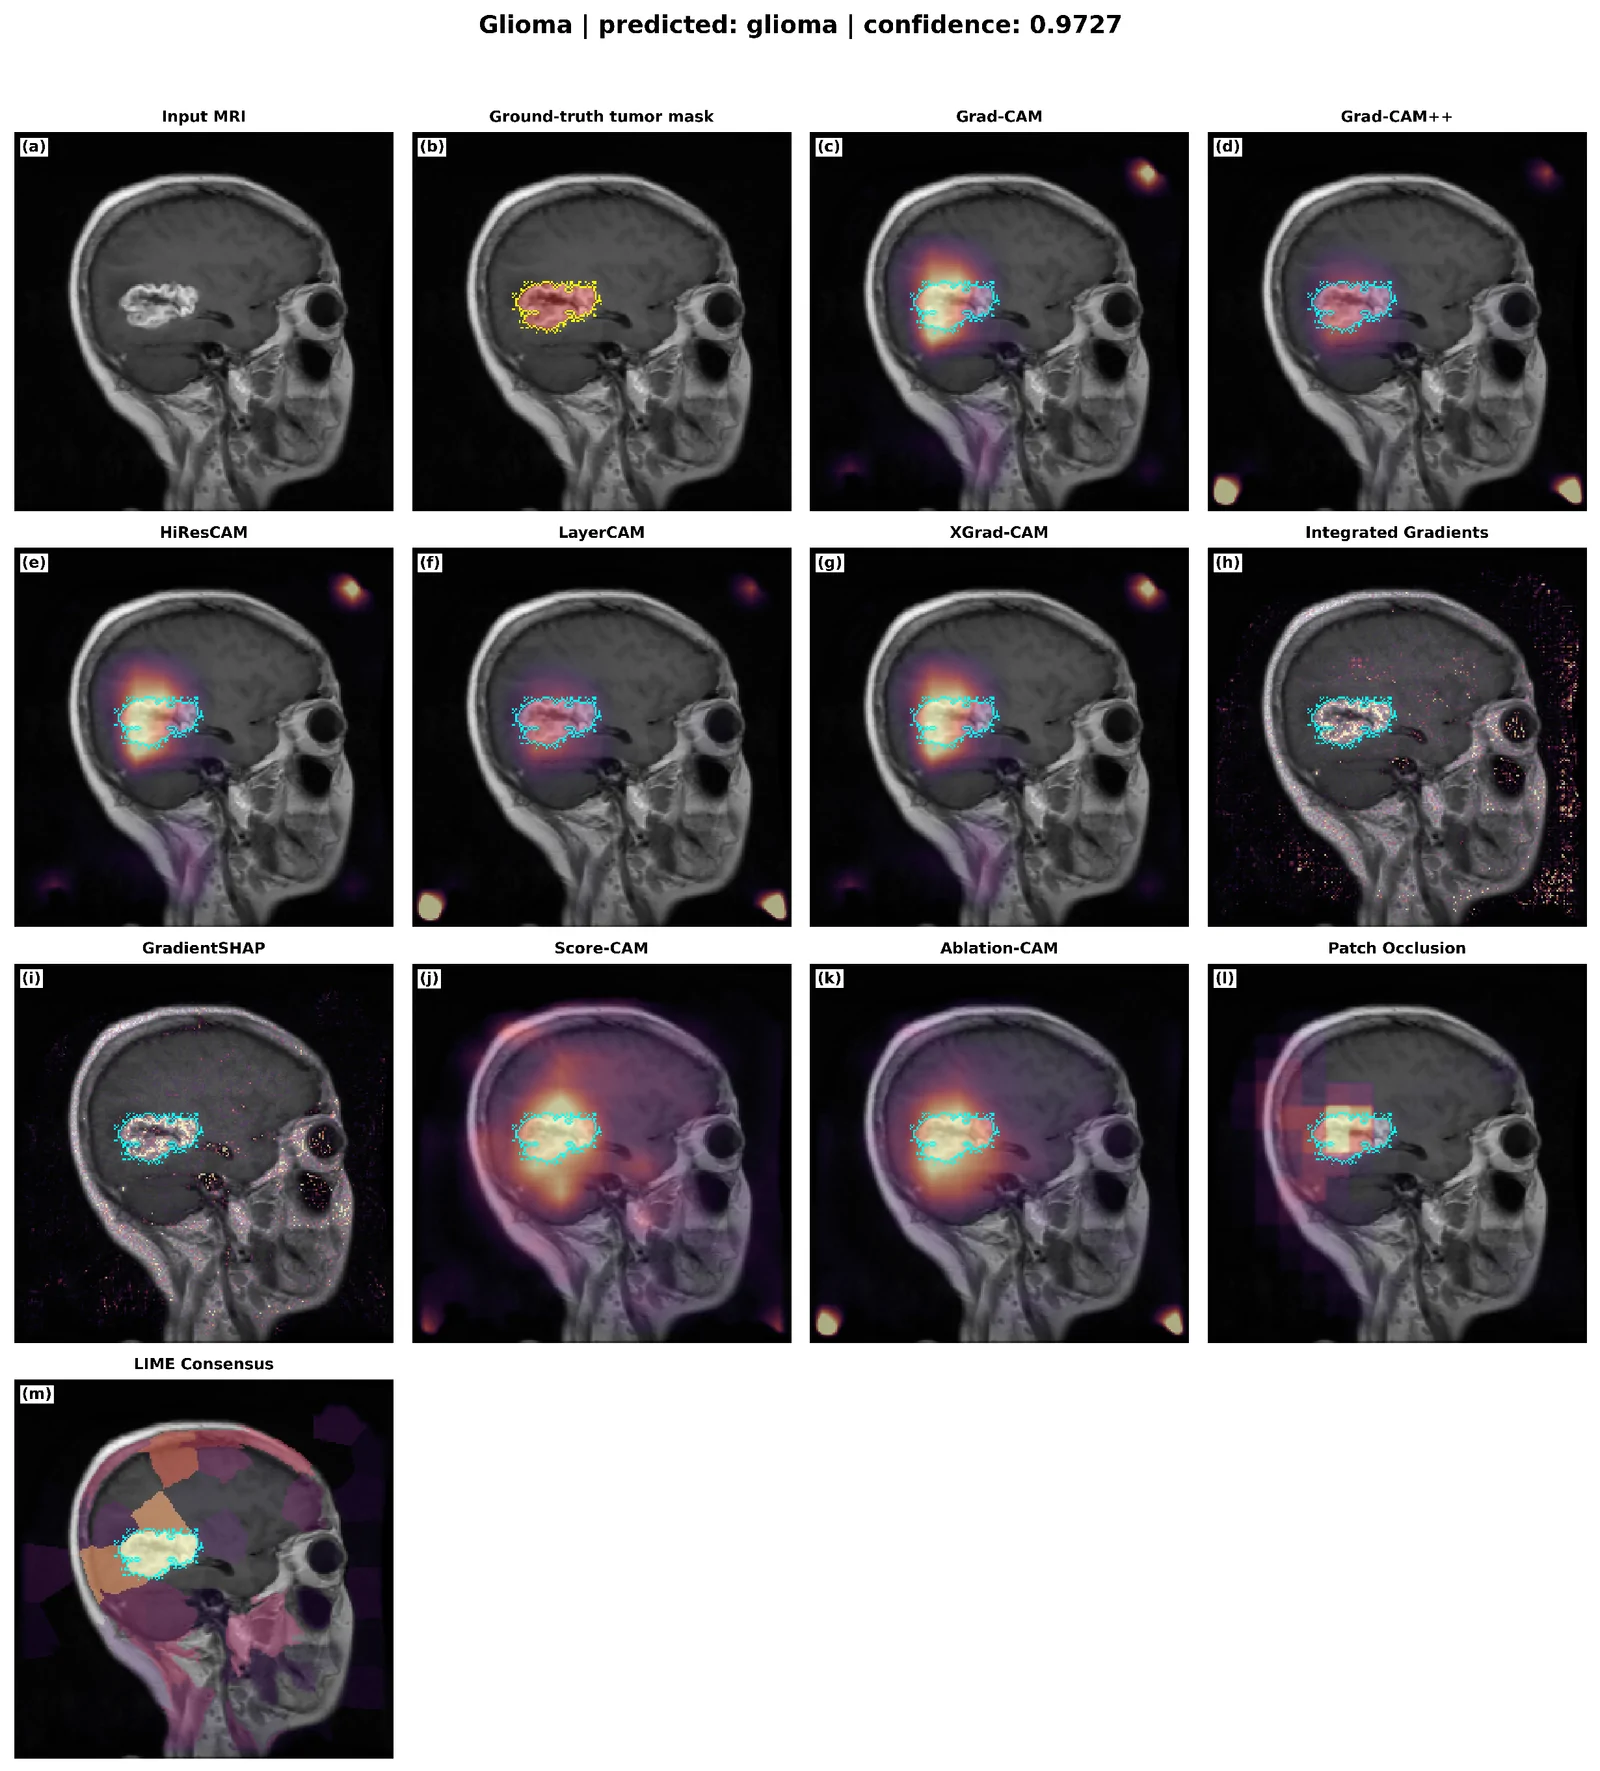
Visualization of all evaluated XAI methods for a glioma MRI image.
